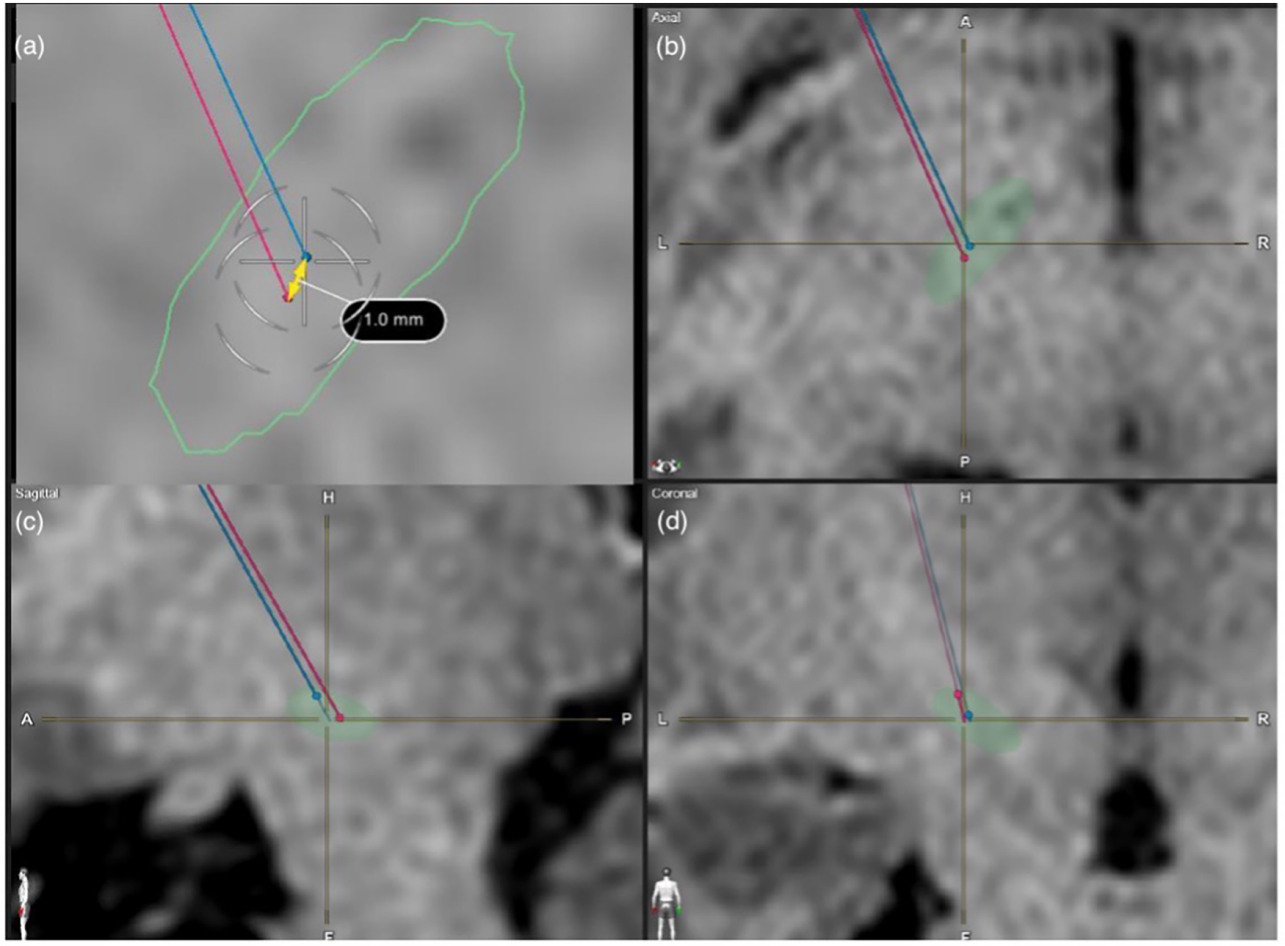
MRI images showcasing a case study whereby the image-based lead trajectory was altered in view of the low EMG-based PTSE threshold. Shown here are T1 sequence MRI Brain images used for lead trajectory planning for STN-based DBS surgery. (a) axial view, (b) enlarged axial view, (c) sagittal view, (d) coronal view. The auto-segmented STN by the Brainlab Elements program was outlined in green. The red trajectory was the original planned image-based trajectory. The blue trajectory was the intra-operatively modified trajectory based on EMG feedback.
